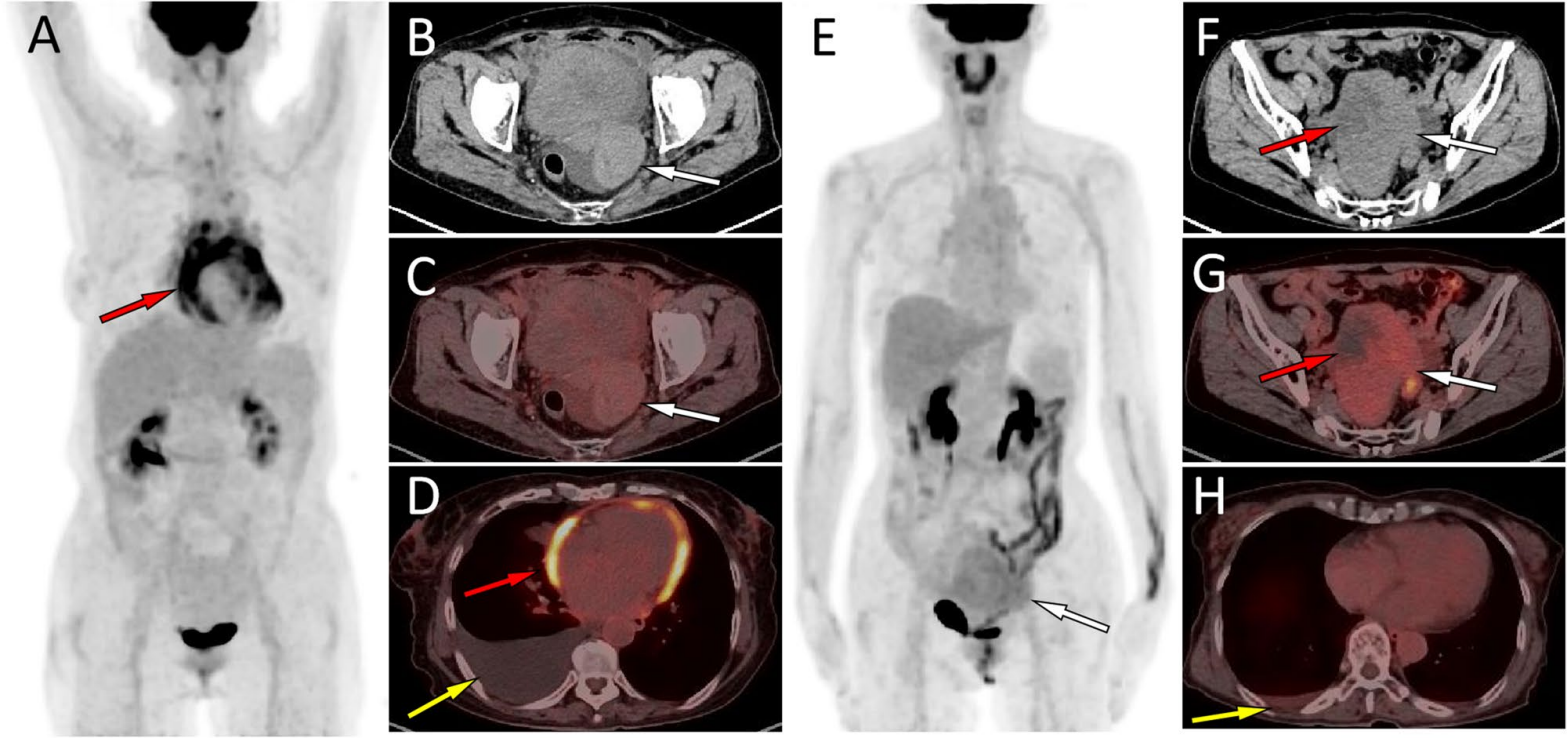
(A–D) Representative ^18^F-FDG PET/CT images of theca fibroma in a 72-year-old woman who sought medical help for two weeks because of pain in the precordial area and difficulty breathing. The maximum intensity projection (MIP, A) showed no significant increase in ^18^F-FDG uptake in the rest of the body except in the pericardial area (red arrow). Axial CT (B, arrow) showed a mass of first order soft tissue density on the left side of the pelvic cavity, and no significant uptake of ^18^F-FDG was observed on the corresponding PET/CT fusion (C, arrow). Axial PET/CT reveals that the lesions shown on the MIP map are pericardial effusions with increased diffuse uptake of ^18^F-FDG (D, red arrow). In addition, an intermediate pleural effusion without increased uptake of ^18^F-FDG was seen in the right thoracic cavity (D, yellow arrow). (E–H) Representative ^18^F-FDG PET/CT images of granulosa cell tumor (adults type) in a 45-year-old woman who sought medical attention for one month due to right chest pain. The MIP map shows a shadow with slightly increased ^18^F-FDG uptake in the lower abdomen (E, arrow). Axial CT (F) showed that the lesion was a soft tissue mass (white arrow) with low density cystic degeneration (red arrow) in the pelvic cavity. The axial PET/CT (G) of the corresponding site showed a mild increase in ^18^F-FDG uptake in the solid part (white arrow) of the tumor, with a SUVmax of 2.4, while no radioactive uptake was observed in the cystic area (red arrow). Axial PET/CT at the chest level showed a small amount of fluid in the right thoracic cavity without radioactive uptake (H, yellow arrow), and the amount of fluid accumulation is significantly lower than the amount of fluid accumulation in the serous cavity caused by serous cavity effusion.
